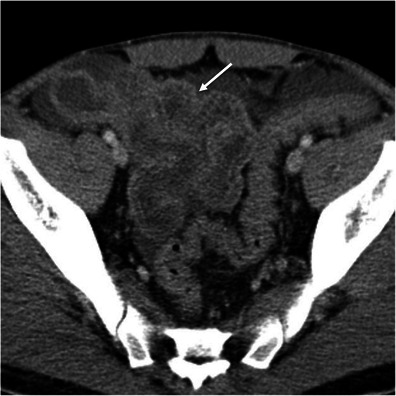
Bowel ischaemia secondary to systemic lupus erythaematosus (LES). Axial contrast-enhanced CT scan shows circumferential thickening of the small bowel loops (*arrows*) with a stratified appearance in a patient with LES presenting with bloody diarrhoea indicating bowel involvement by the vasculitis
